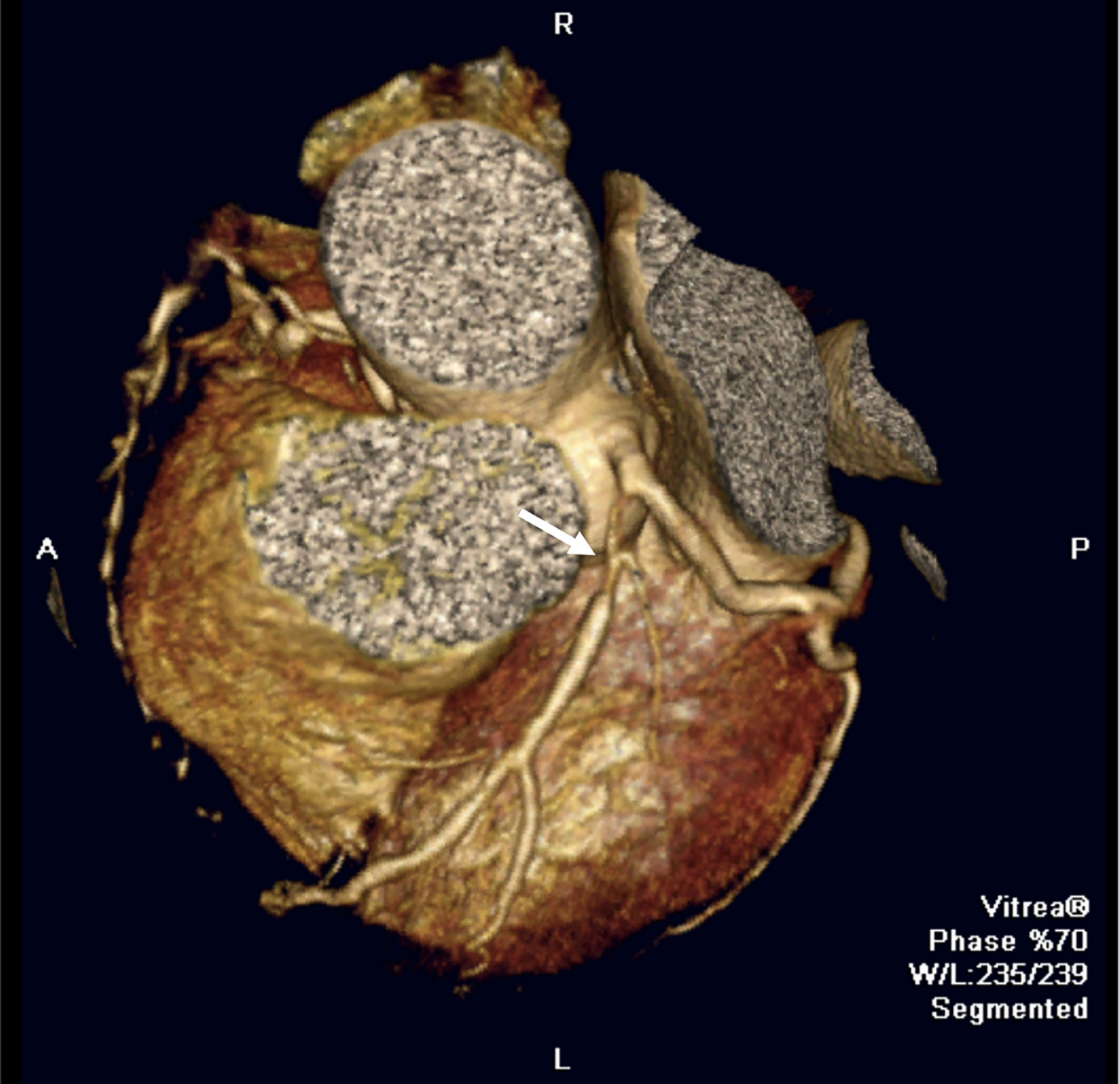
Cardiovascular manifestations in SLE. Coronary computed tomography image showing a severe stenotic lesion in the proximal segment of the left anterior descending coronary artery (white arrow) in a SLE patient.
